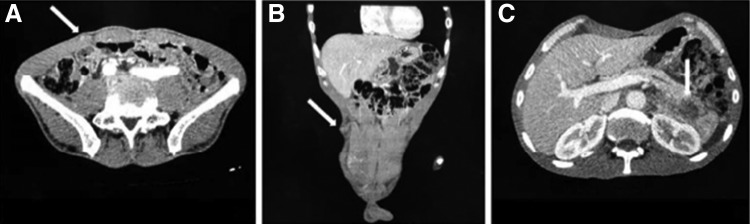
(A, B) Axial CT scan demonstrating a hypodense nodular lesion with barely defined contours located on the abdominal wall near the upper port site (*arrow*). (C) CT scan demonstrates a heterogeneous mass adjacent to the pancreatic tail (*arrow*).
